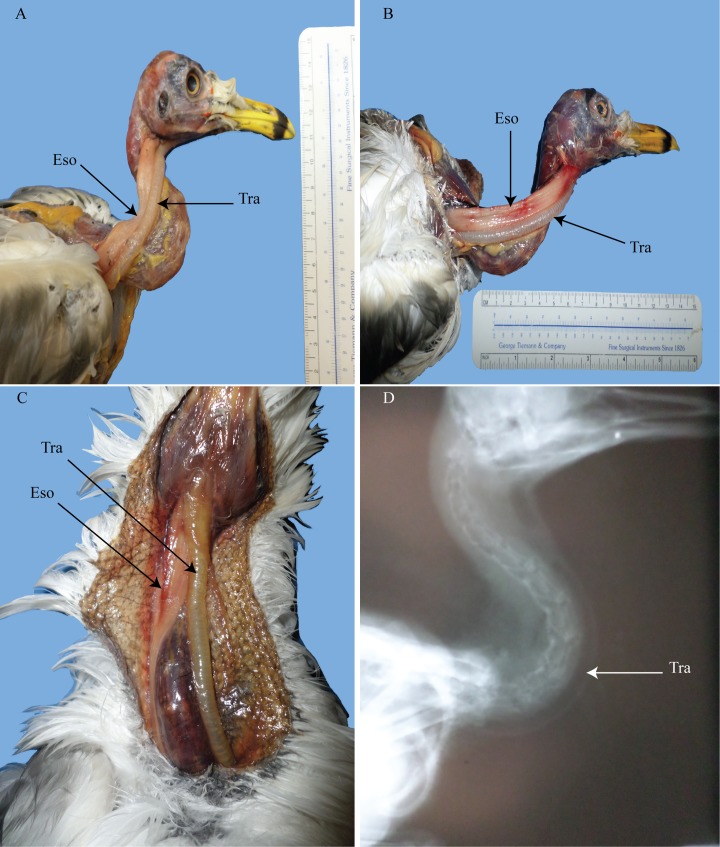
Larus delawarensis*. (A) lateral view of ring-billed gull. (B) lateral view of ring-billed gull. (C) ventral view of ring-billed gull showing the trachea on the left side of the neck. (D) radiograph of the gull from C with a left ventrolateral trachea.
